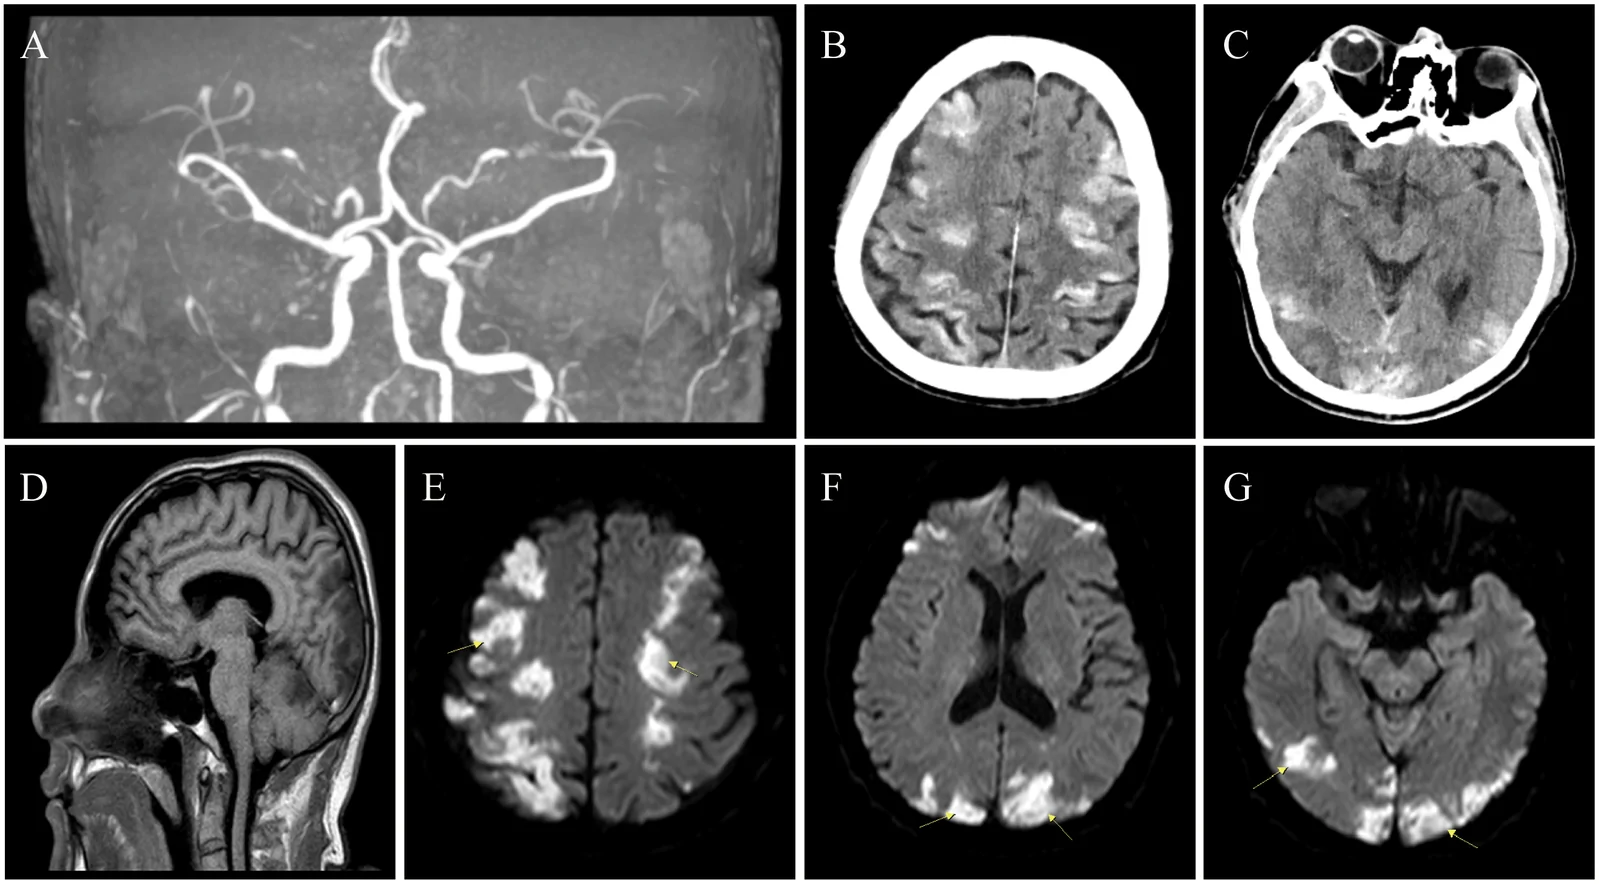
Brain imaging after intraoperative neurological deterioration. (A) MRA showing no definite major intracranial arterial occlusion. (B, C) Immediate cranial CT showing multiple hyperdense intracranial lesions; virtual non-contrast imaging supported iodized-oil deposition. (D) Sagittal MR image. (E–G) Diffusion-weighted MRI showing multifocal acute ischemic lesions in bilateral supratentorial and infratentorial regions.
